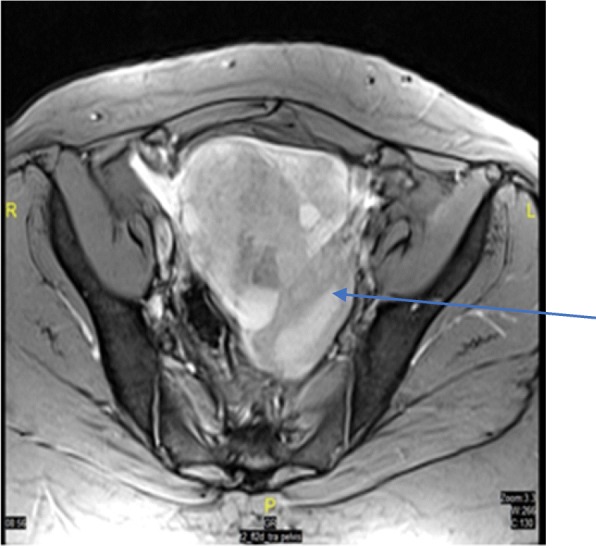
Transverse T2 MRI section. The section shows well-defined complex lesion (arrow) with solid and cystic contents in the pelvis, extending on either side of the midline reaching to both sides of adnexa and measuring 13 × 9.4 × 8.1 cm. Ovaries are not seen separately from the lesion. Uterus shows mild deviation to the left side due to pressure effect from the mass. No obvious lymph nodes or signs of metastasis
